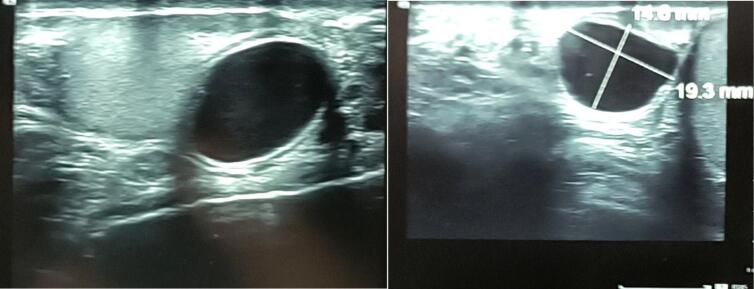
Ultrasound Imaging of a Hemorrhagic Epididymal Cyst.
